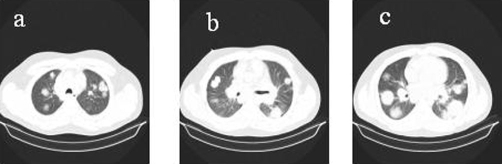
Non contrast chest CT scan, pulmonary window: multiple bilateral masses, in a patchy distribution, of increased density and lobulated contour. Some have a ground-glass opacity. Associated mediastinal lymphadenopathy (bilateral hilar, subcarinal, paratracheal) is noted, which is better appreciated using this window.
